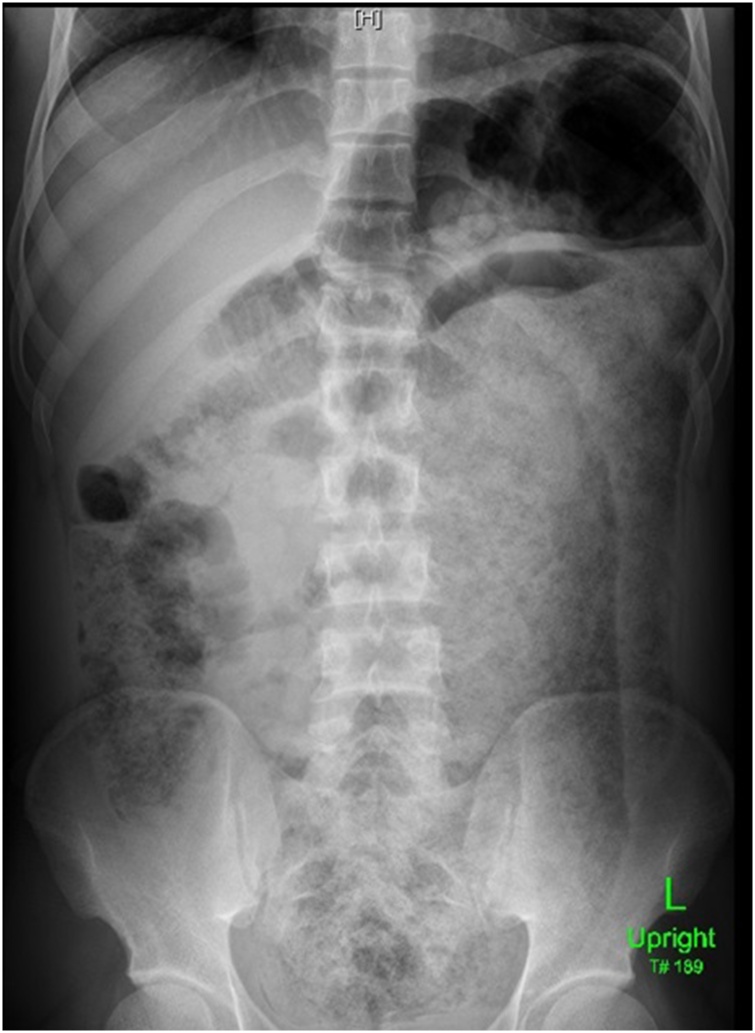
Plain erect abdominal X-ray showing huge fecal loading of the whole colon.
